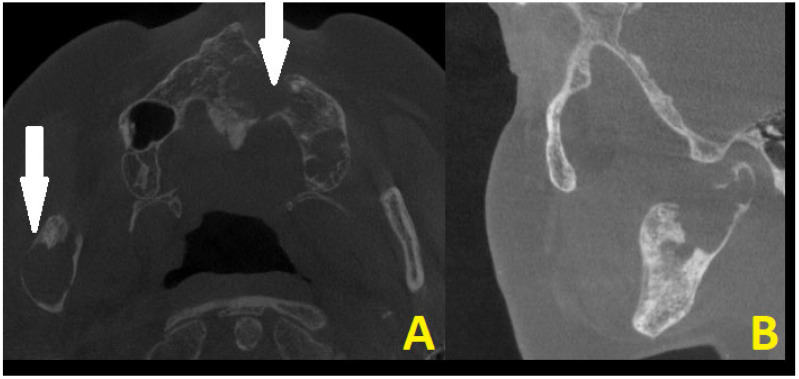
CBCT scans in axial projection (A) with maxillary destruction and sagittal (B) visualization of the cystic destruction of the right condylar process and condylar head. Cystic destruction of the left maxillary bone with an extra bone spread towards the lip and nasal cavity, and right mandibular ramus swelling with proliferation towards the condylar head is noted (white arrows). In surgical planning, the condition of the bone is very important for a good radiological bone evaluation, as well as for cortical bone estimation. Secondly, each surgery must maintain each patient’s quality of life (QOL). In the case of a 69-year-old patient, the most troublesome factors were related to an inability to fully open their mouth, the occurrence of transient pain, inflammation, the presence of right preauricular swelling, and the deterioration of chewing and biting ability. The first surgery step was used to remove all small lesions, fill them with allogenic bone grafts, and perform a right-sided coronoidectomy with cyst removal and decompression to prepare the right condyle for the second stage of surgery. The main troublesome course of surgery included the necessity to remove part of the bone TMJ structures affected by the disease and reconstruct the TMJ for the best possible outcome to enable the elderly lady to be able to properly open her mouth, eat, and speak. Because of this patient’s economic means and a great fear of a total TMJ artificial endoprosthesis, a decision was made to reduce both the costs and scope of surgery, meaning a reduction in the usage of many materials and foreign body components from a custom-made TMJ endoprosthesis. Secondly, since the OKC did not do any destruction within the condylar fossa, the articular disc and TMJ joint were healthy, so the usage of an acetabulum, namely the glenoid fossa alloplastic component, was not necessary. Planning a good CBCT is enough to plan the scope of bone resection and removal of all polycystic lesions from the right mandibular head, condyle, and part of the ramus. Because of the locally aggressive character of OKC, their extracortical spread, polycystic occurrence, and joint manifestation, a decision was made for more aggressive surgery with immediate joint reconstruction in the second stage of surgery when mouth opening was improved. Both surgeons (K.N., K.Ł.) decided to improve the overall mouth opening and excise all advanced aggressive OKC lesions at first, and then decide on the scope for TMJ surgery to improve occlusion and bite, and prepare for further patients’ prosthodontic treatment. No necessity for additional CT, MRI, or similar studies was needed.
